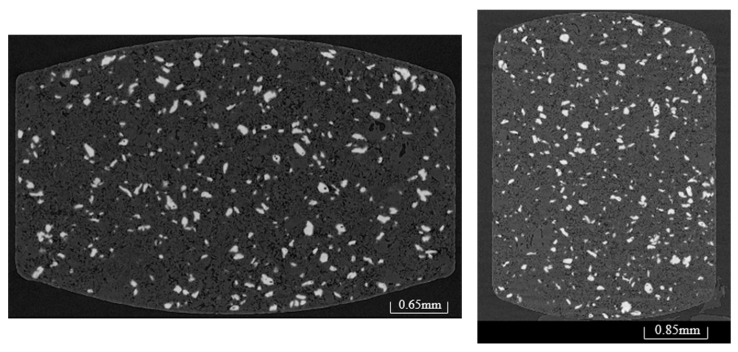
X-ray CT images of the inside of Dex 5 mg coated tablets (left) and Dex 2.5 mg coated tablets (right). The white dots indicate DEX.
